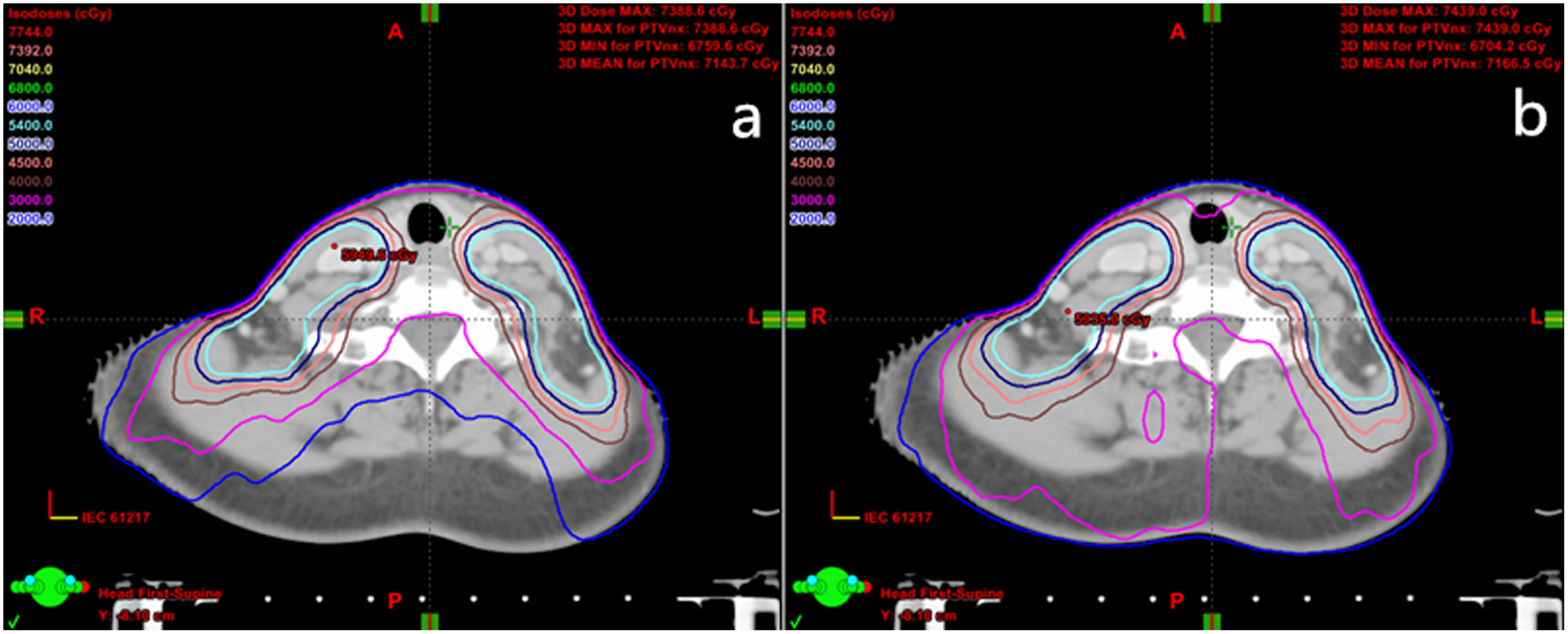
Isodose distributions of the large-field volumetric arc therapy (LF-VMAT) and fixed-jaw VMAT (FJ-VMAT) plans. (a) The FJ-VMAT plans. (b) The LF-VMAT plans. Eleven dose lines of 20–77 Gy from the outside to the inside of each CT image.
